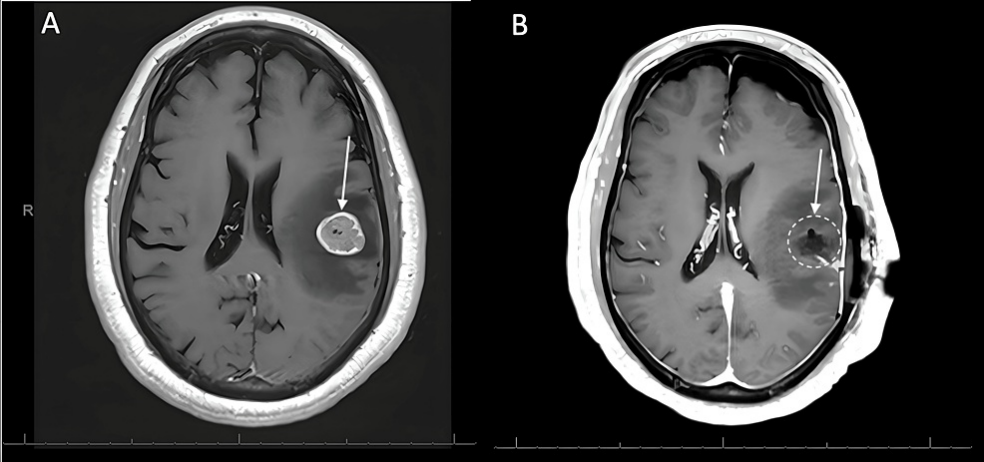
(A) Preoperative axial CT scan showing a frontoparietal mass consistent with metastasis. (B) Postoperative axial CT scan showing resection cavity.
